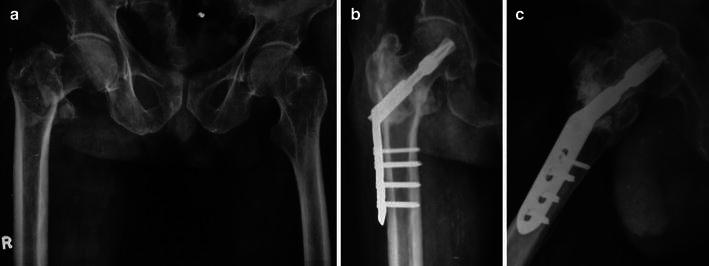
a Preoperative anteroposterior radiograph in a 82-year-old male showing 31A2.2 pertrochanteric fracture. b Follow-up anteroposterior radiograph of the same patient showing union. c Follow-up lateral radiograph of the same patient showing union
